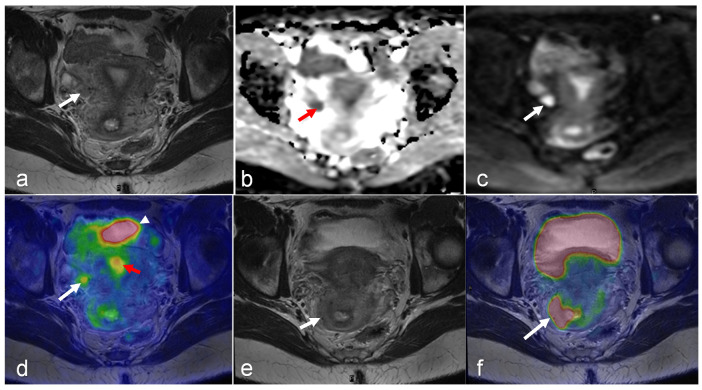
A 22-year-old woman with stage IB2 cervical cancer clinically or stage IIIC1 cancer as determined via PET/MRI due to a metastatic lymph node. The metastatic lymph node (arrow) is hypointense on the axial T2-weighted MRI (a); low signal on the ADC map (b); high signal on the DWI (c), with FDG uptake on fused PET/MRI (d) images. Physiologic FDG uptake in bladder (arrowheads in (d)) and endometrium (red arrow in (d)) can be seen. Both axial T2-wighted MRI (e) and axial-fused PET/MRI (f) show the cervical tumor with a suspicious irregular right margin (arrow in (e)) with pathologic FDG uptake interpreted as parametrial invasion (arrow in (f)). (Courtesy of Elisabeth Hedlund, MD, Håkan Ahlström, MD, and Björg Jónsdóttir, MD, PhD, Uppsala University, Uppsala, Sweden).
